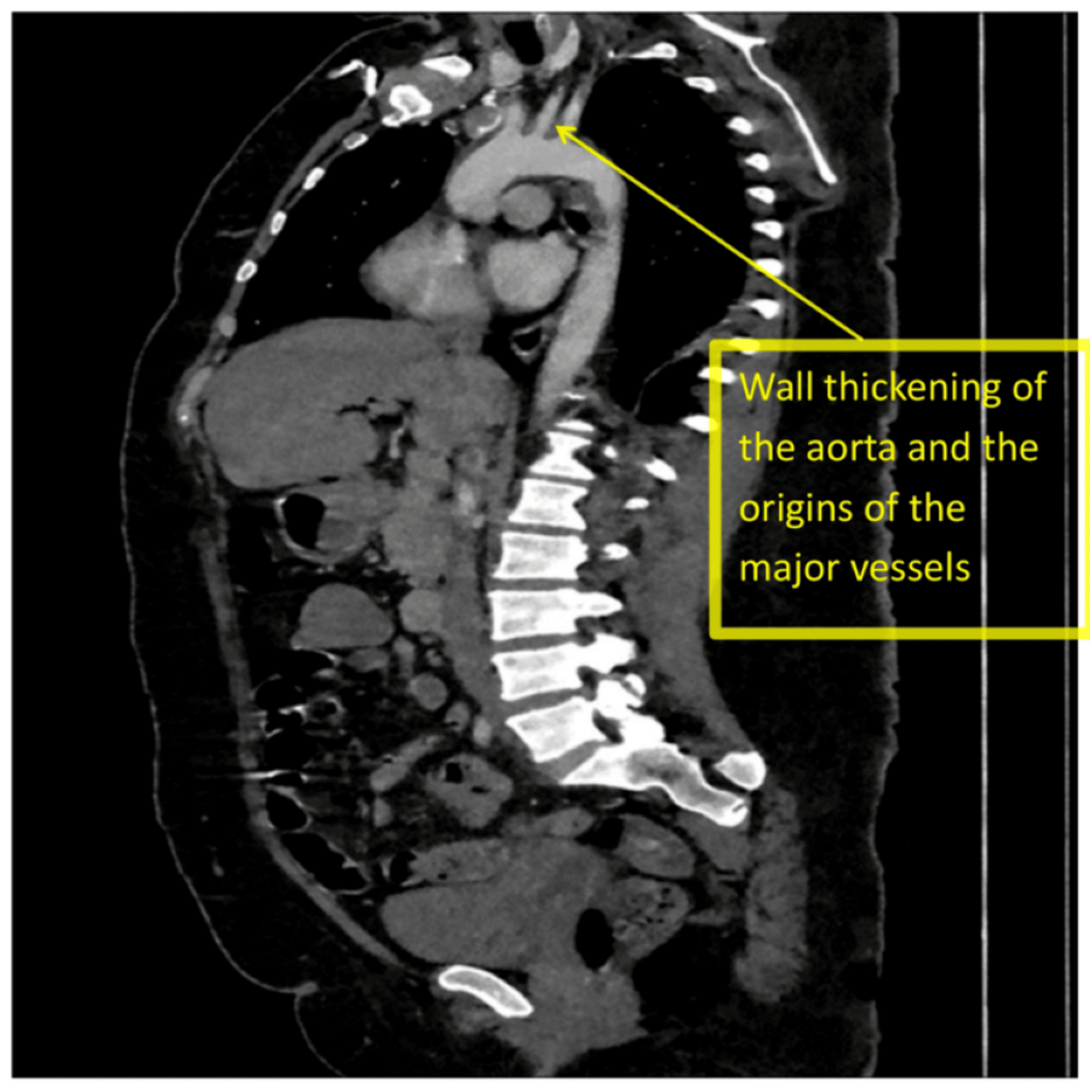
Sagittal section of CT aortography showing wall thickening of the aorta and the origins of the left subclavian, common carotid, and right brachiocephalic artery
